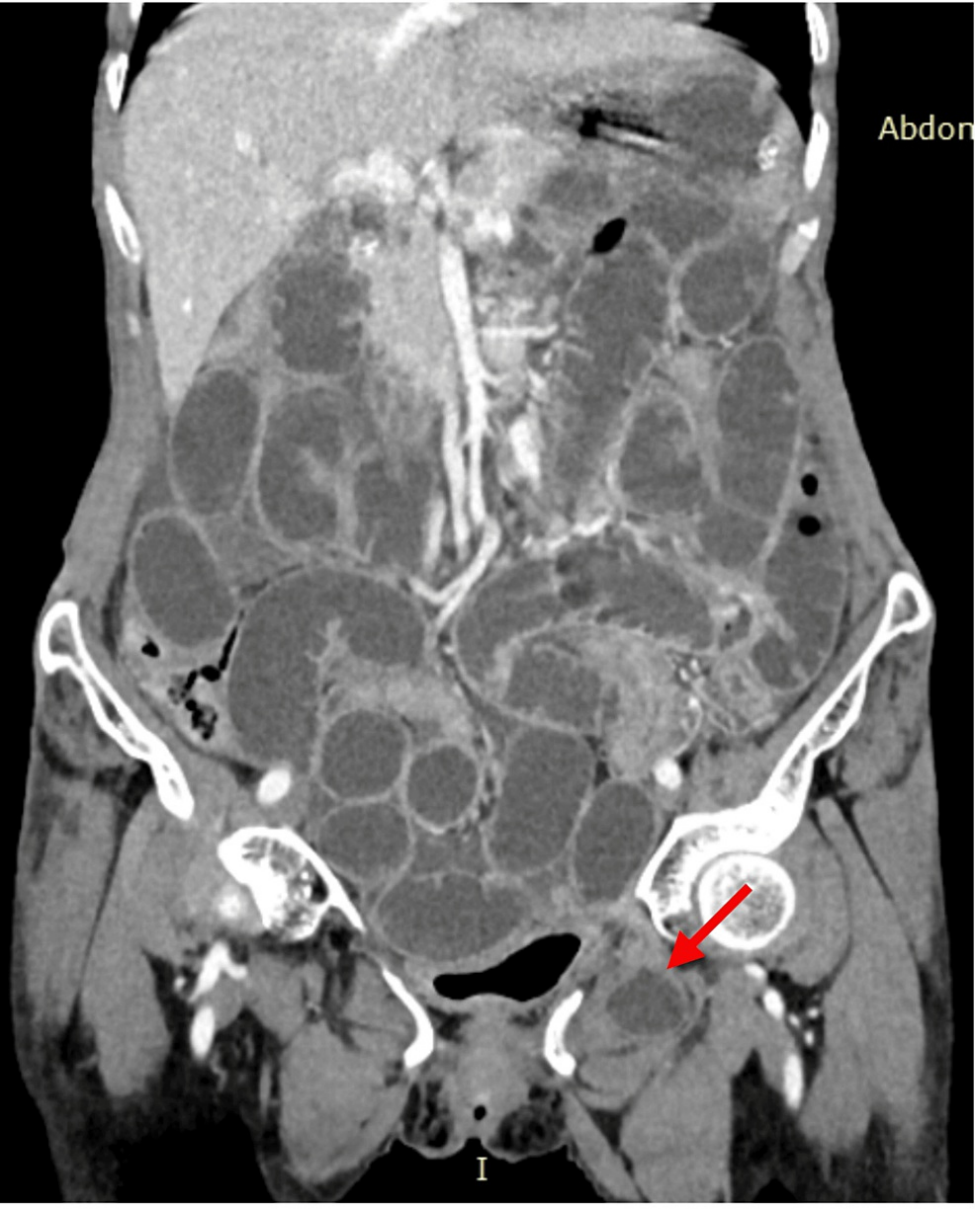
CT scan showing left-sided obturator hernia Arrow indicates the herniated small bowel loop through the obturator foramen
